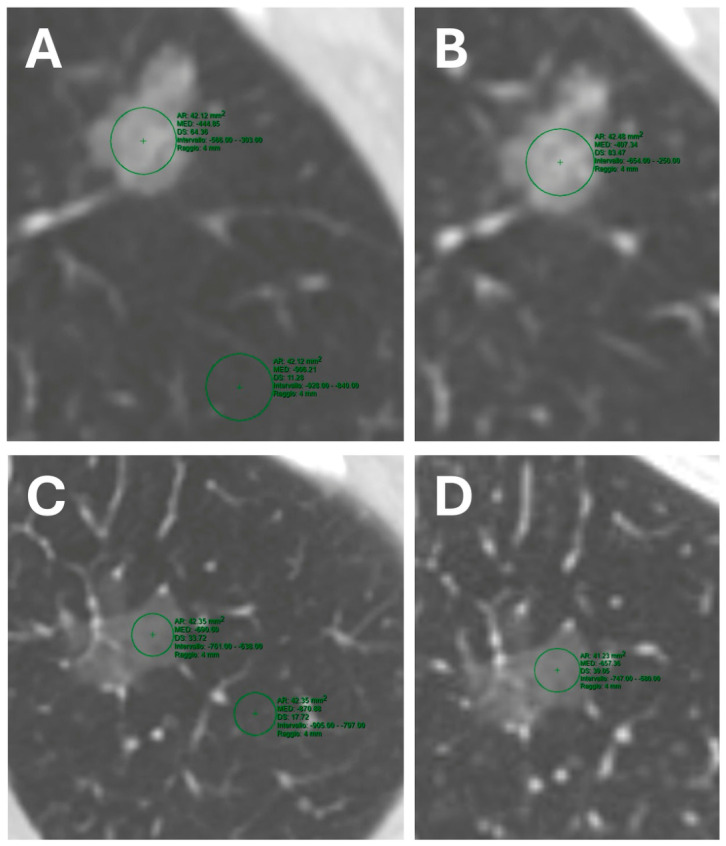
Pure ground-glass opacity (pGGO) and healthy lung density measurements on preoperative computed tomography (CT) scan, from which the “difference CT value” and “relative attenuation” are derived. The images shown are from the same patient, who presented with two synchronous pGGOs. (A) Unenhanced CT of an invasive adenocarcinoma (IAC). Two 4-mm-radius regions of interest were placed on the tumor (−444.85 HU) and on adjacent healthy lung parenchyma at least 10 mm away (−906.21 HU). (B) Post-contrast arterial-phase CT acquisition of the same lesion, here displaying a higher density (−407.34 HU). (C) Unenhanced CT of an adenocarcinoma in situ (AIS). The tumoral tissue density is −690.60 HU, lower than that of the other nodule, while the surrounding healthy tissue density is −870.88 HU. (D) The same AIS, in the post-contrast CT acquisition, shows an increased attenuation of −657.36 HU.
